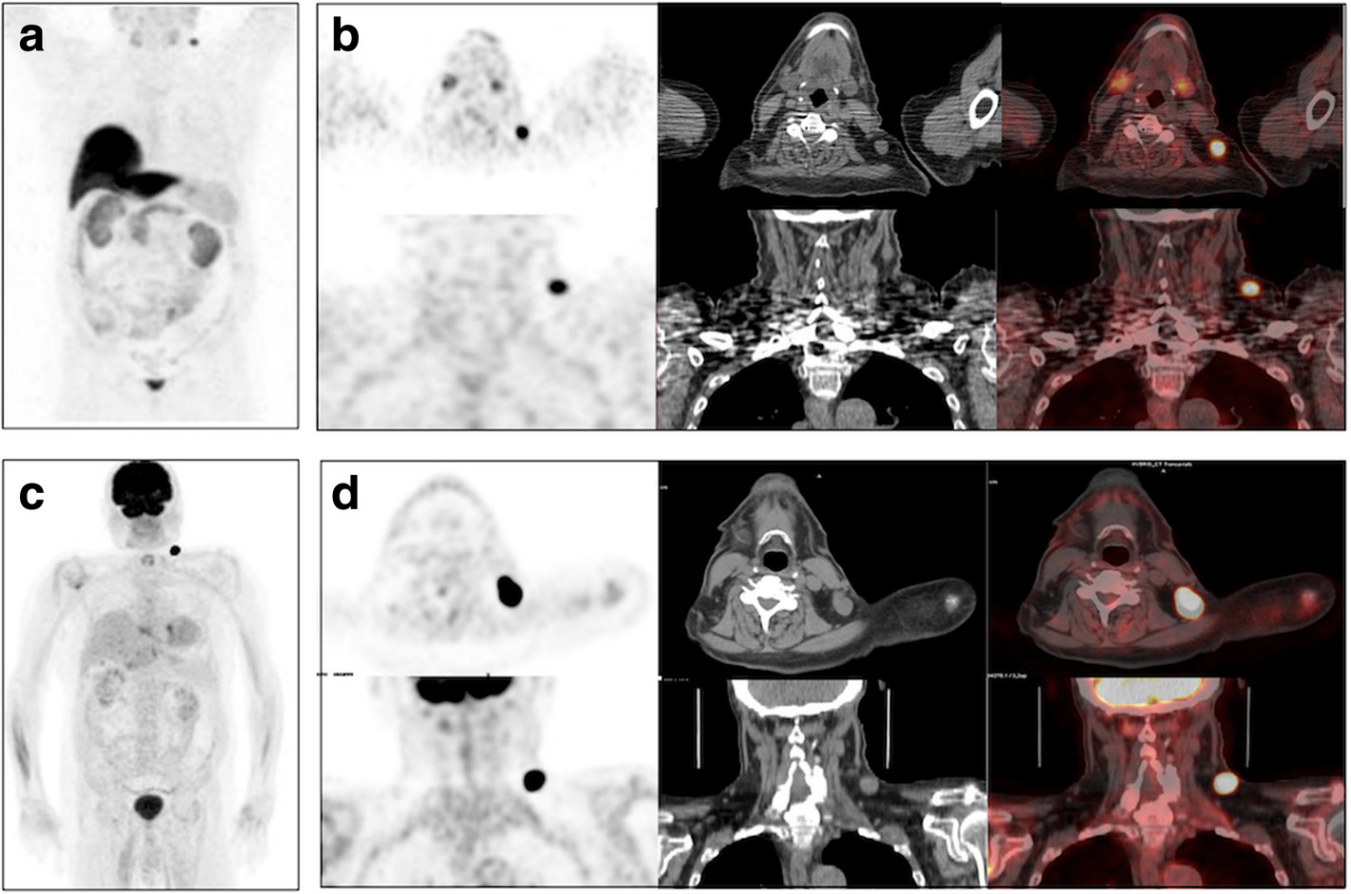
Case #4, 80-yr old man treated with radical prostatectomy for prostate cancer, presenting with progressive PSA raise. MIP (a), axial and coronal (b) [^18^F]fluorocholine PET/CT images show an area of intense [^18^F]fluorocholine uptake in a left supraclavicular lymph node. The patient underwent a [^18^F]FDG-PET/CT, that confirms the finding as shown by MIP images (c), transaxial and coronal images (d). Biopsy diagnosed a nodal metastases from melanoma
